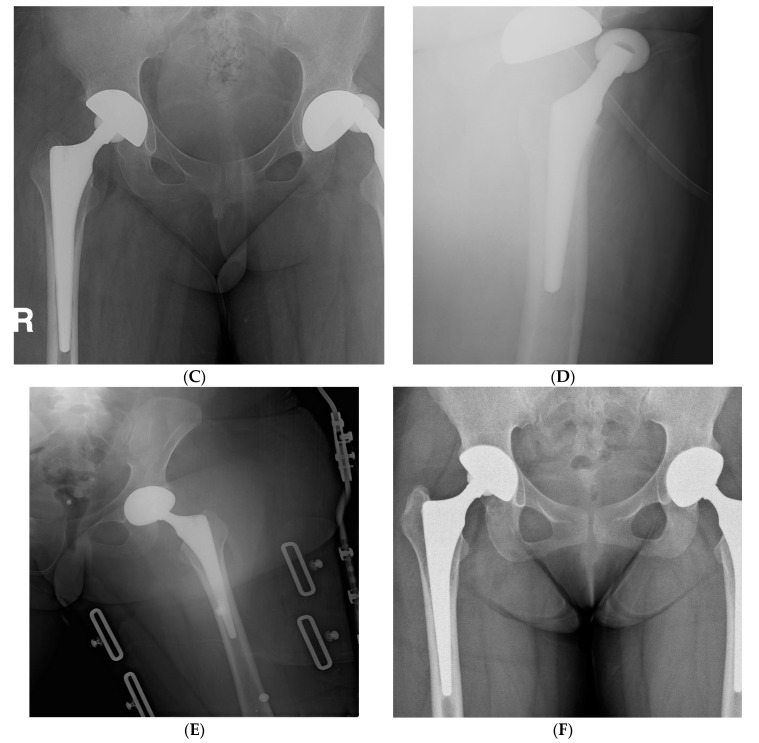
(A,B) Preoperative and postoperative hip radiographs of a 33-year-old woman with cognitive dysfunction and visual disturbance after removal of recurrent craniopharyngioma. She underwent simultaneous bilateral THA due to ONFH. (C,D) Radiographs of the same woman on the 15th postoperative day, when she visited the emergency department due to severe pain in the left hip. Anteroposterior and lateral images demonstrated anterior hip dislocation. (E) Hip radiograph after performing closed reduction followed by abduction brace application under general anesthesia. (F) Eight-year postoperative radiograph showed stable implant fixations.
